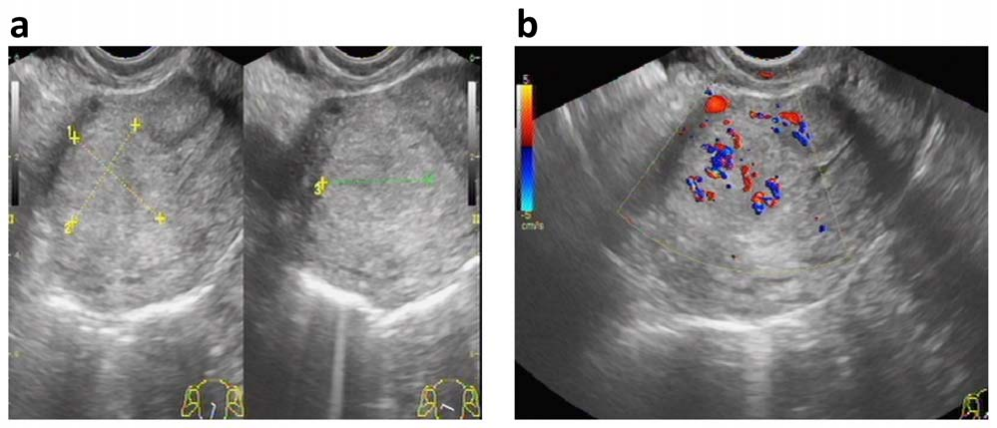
Ultrasonographic characteristics of PSTT. (a) On gray-scale image, the lesion was located in the anterior wall of uterus, showing the hyperechogenic solid mass with unclear border (between cursors). (b) On Color Doppler, the abundant Doppler signals from blood flow presented within the boundary of tumor rather than at the periphery, which is named as “non-peripheral Doppler signal”.
